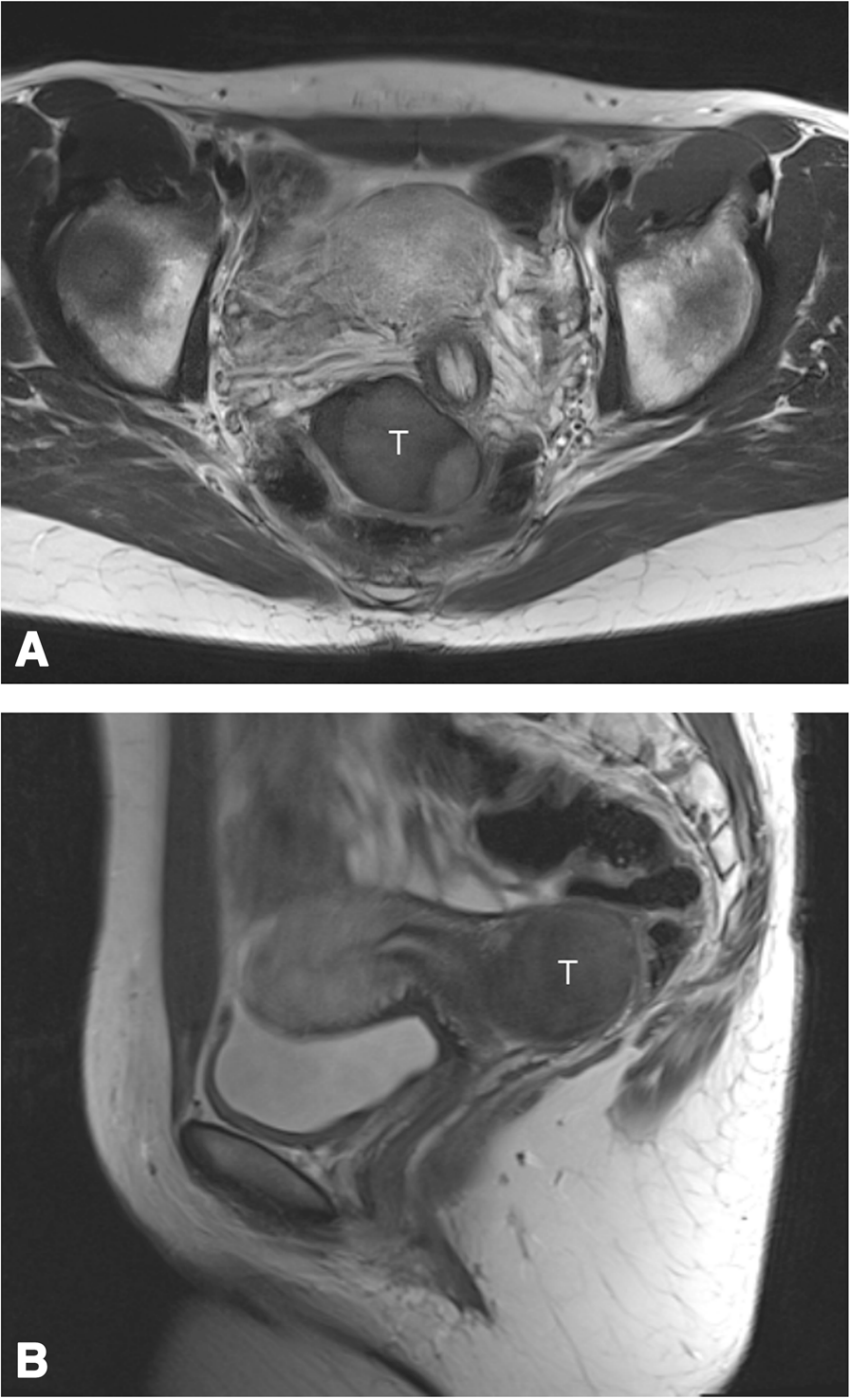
Coronal (a) and sagittal (b) T2-weighted magnetic resonance images obtained preoperatively show a homogeneous solid mass (T) displacing the cervix at *left* (seen best in coronal view)
